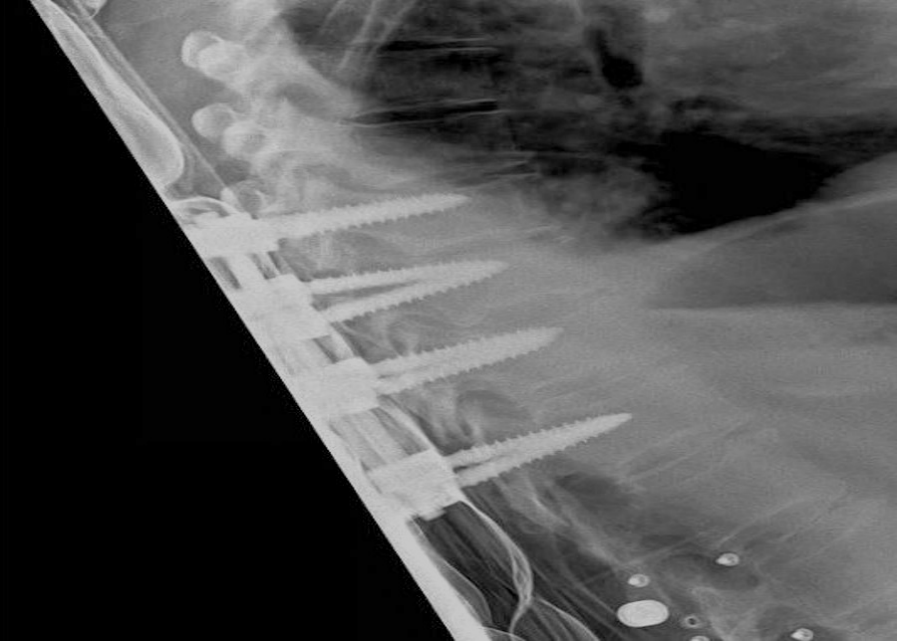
Lateral x-ray image demonstrating trajectories of the pedicle screws.
